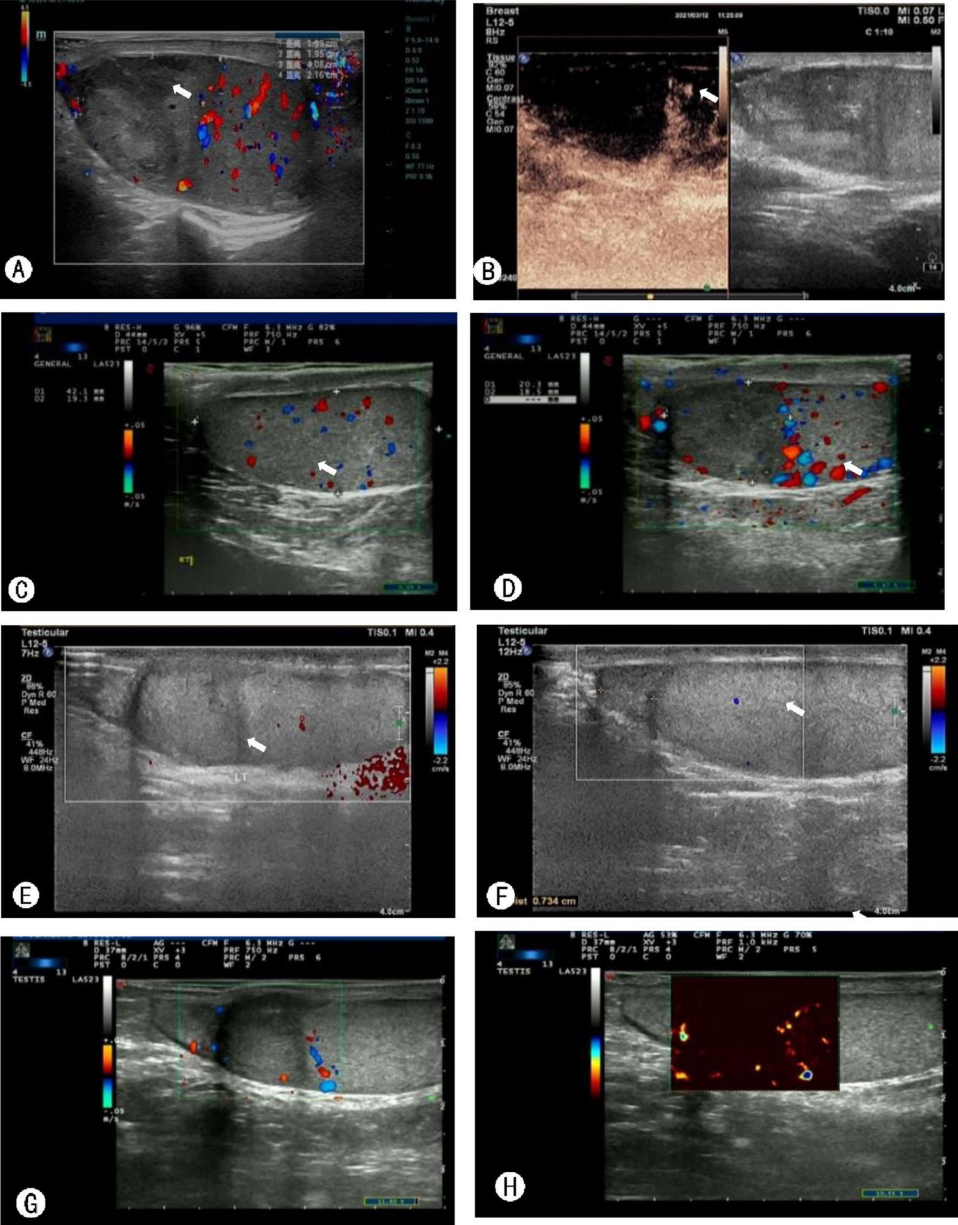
Ultrasonography was performed after infarction, revealing uneven localized heterogeneous echogenicity with no internal blood flow signal in the upper pole of the left testis, measuring 24 mm * 26 mm (A). The upper part of the left testicle showed an avascular area (B). One week after the infarction, the ultrasound showed that the echogenicity of the upper half of the left testis was reduced, measuring 20 mm * 19 mm (C), and several blood flow signals (D) were seen at its edge. Three weeks after the infarction, the ultrasound showed that the echogenicity of the upper half of the left testis was reduced, measuring 15 mm * 16 mm (E), and several punctate foci with strong echogenicity (F) were observed. Five weeks after infarction, the ultrasound revealed heterogeneous localized echogenicity in the left testis, measuring 14 mm * 15 mm (G), and enhanced signals (H) appeared in the infarct after contrast
